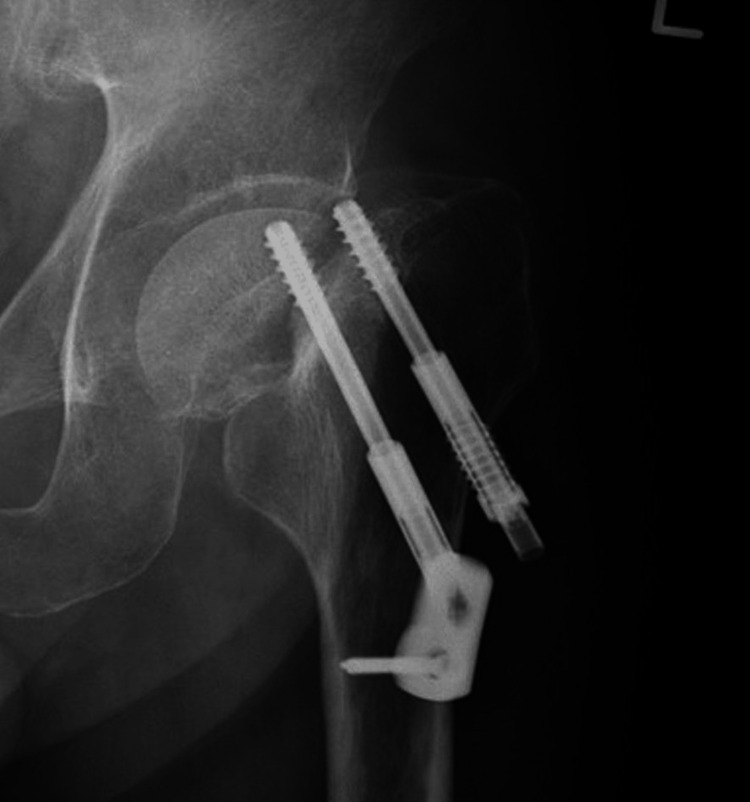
X-ray two years after surgery: fixation failure (cut out) occurred
